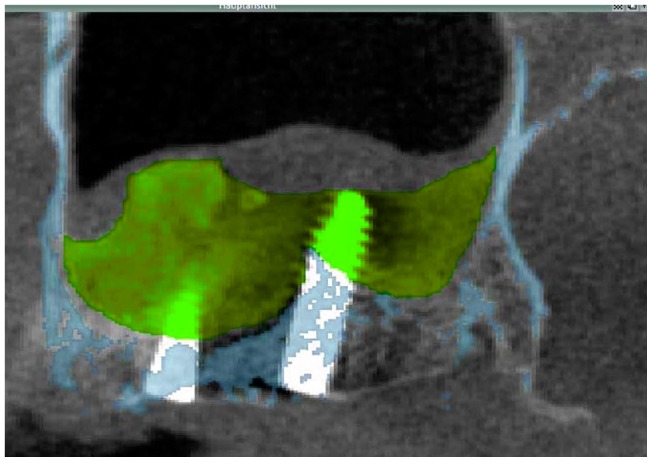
Two-dimensional radiographic image generated from the tertiary cone-beam CT acquired at implant placement 6 months after SFA showing of a cross section through grafted area after the 6 months healing period (green) as well as the osseous anatomical structures of the native sinus floor (blue). This image was generated by merging the data sets of the primary and tertiary cone-beam CTs in combination with superimposing the image of the secondary cone beam CT (light grey). Sagittal view of the left-sided maxillary sinus, patient 5.
